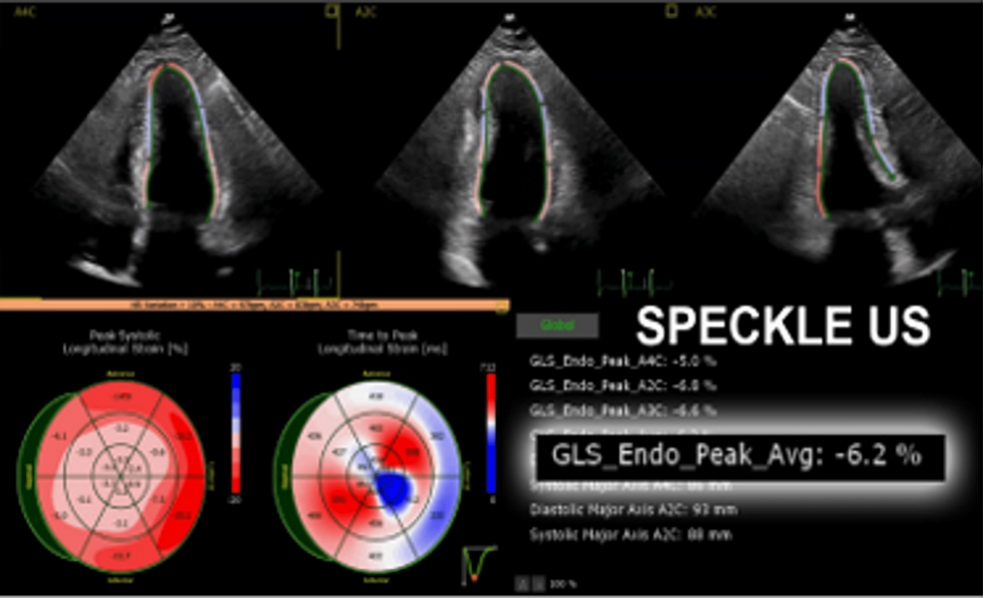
Speckle Echocardiography of the Patient’s Heart Left ventricular speckle echocardiography shows a decreased GLS of -6.2%. GLS, global longitudinal strain.
